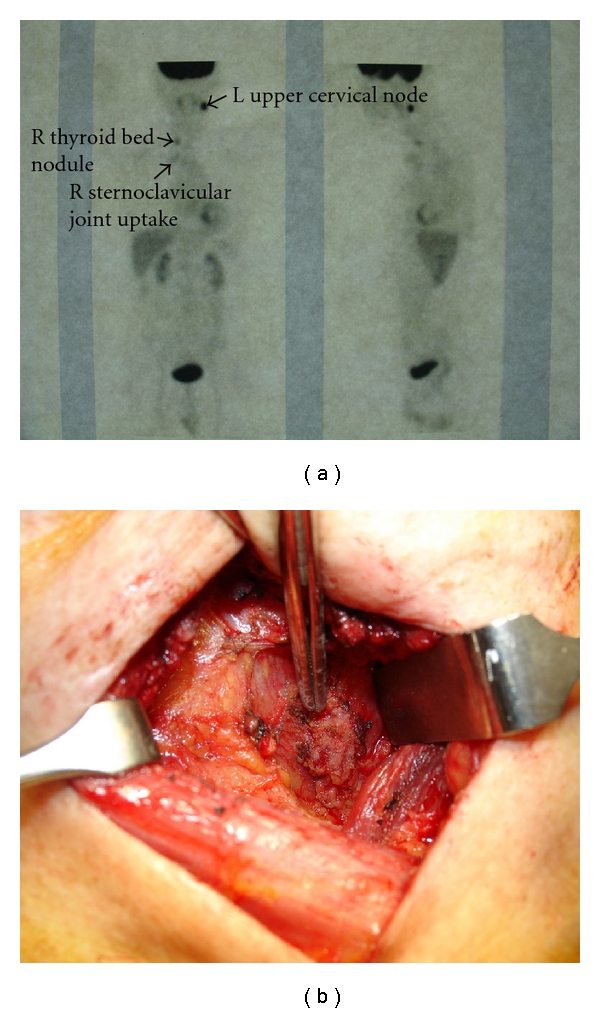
18F-FDG-PET of a 73-year-old man with an 8-year history of papillary thyroid carcinoma. The serum thyroglobulin was elevated at 66 ug/L (normal < 55 ug/L), but both diagnostic whole-body ^131^I scan and ultrasound were negative for recurrence. (a) FDG-PET revealed hypermetabolic uptakes in left cervical lymph node, right thyroid bed, and right sternoclavicular joint area. (b) An operative picture confirming the presence of tumor recurrence at the right thyroid bed (as pointed by the tip of the forceps).
